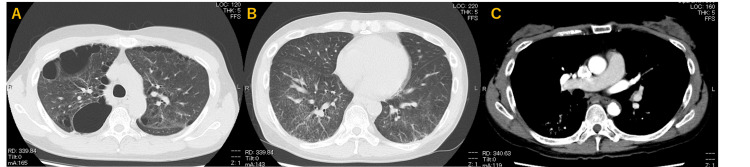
Plain CT showed a bulla at the periphery of the bilateral upper lobes (A), and ground-glass opacities extending to both lobes (B). Contrast-enhanced CT showed no enlargement of the pulmonary arteries or thrombus in the pulmonary arteries (C).
